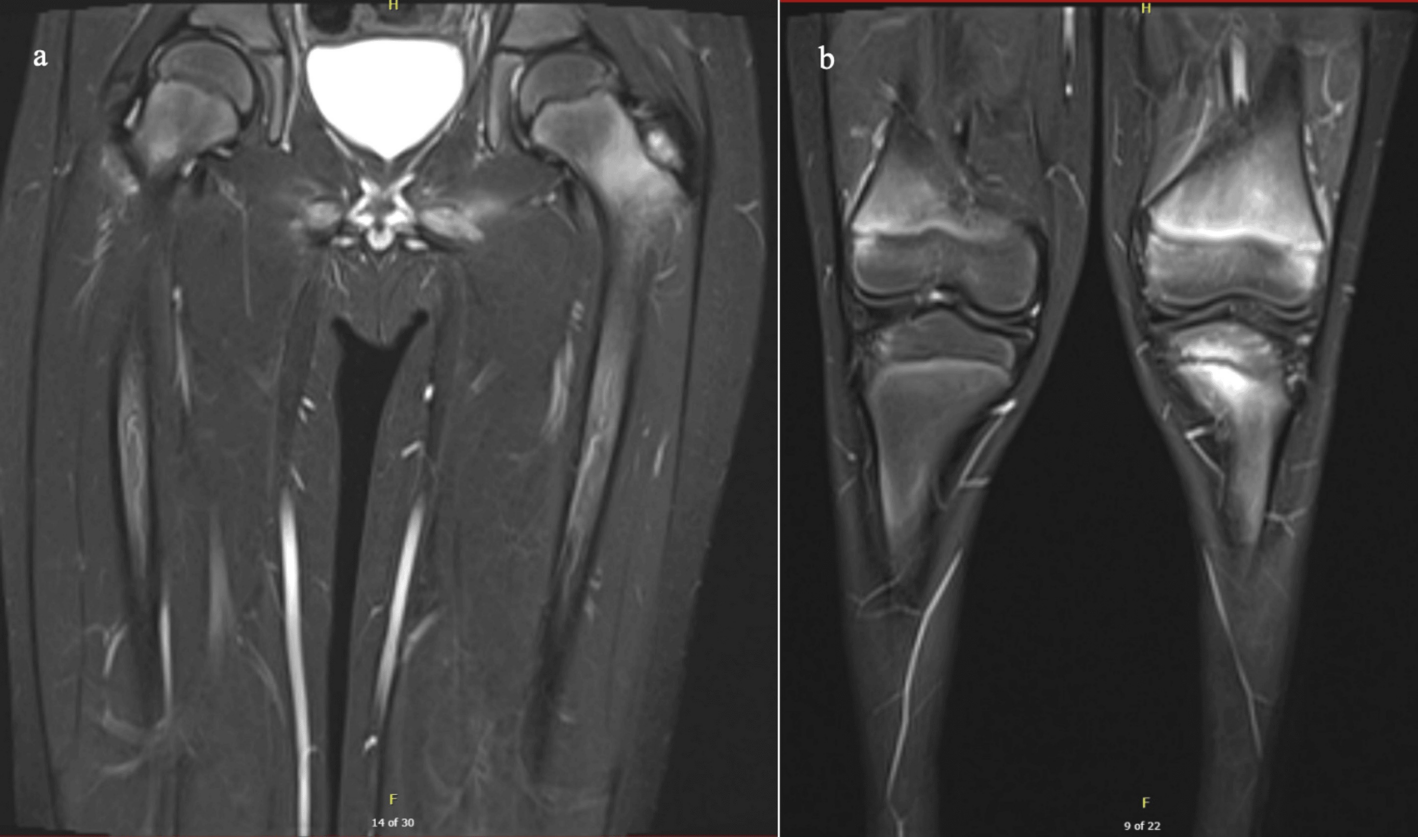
Whole body MRI showing edema-like marrow signal noted throughout the tibias and femurs
